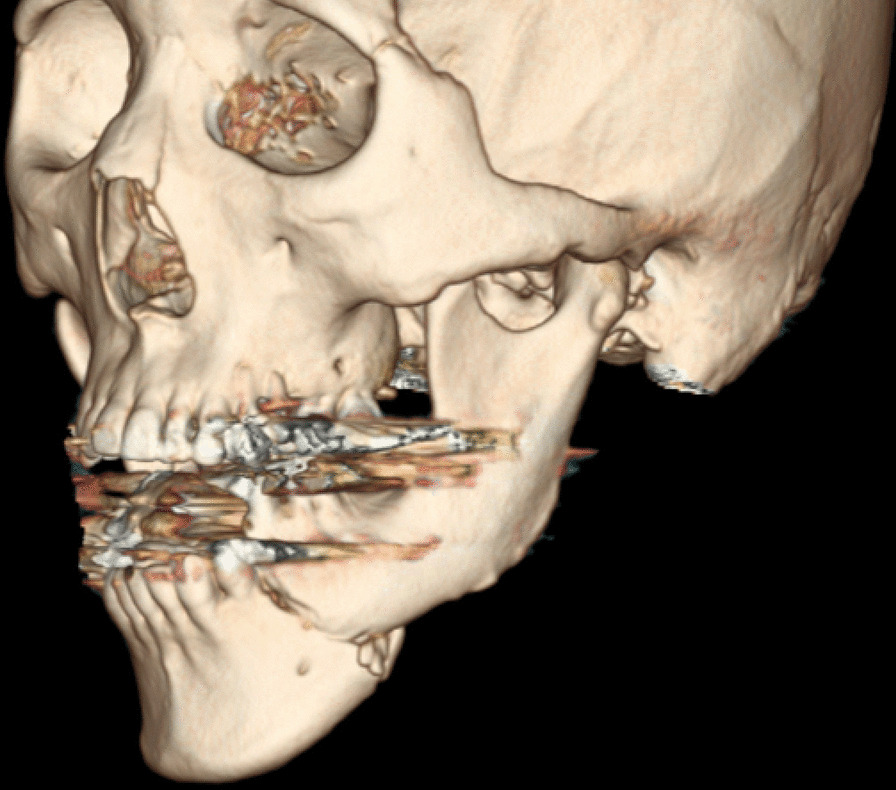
Three-dimensional computed tomography findings obtained during the initial visit showed fracture characterized by severe deviation of the left mandibular body
